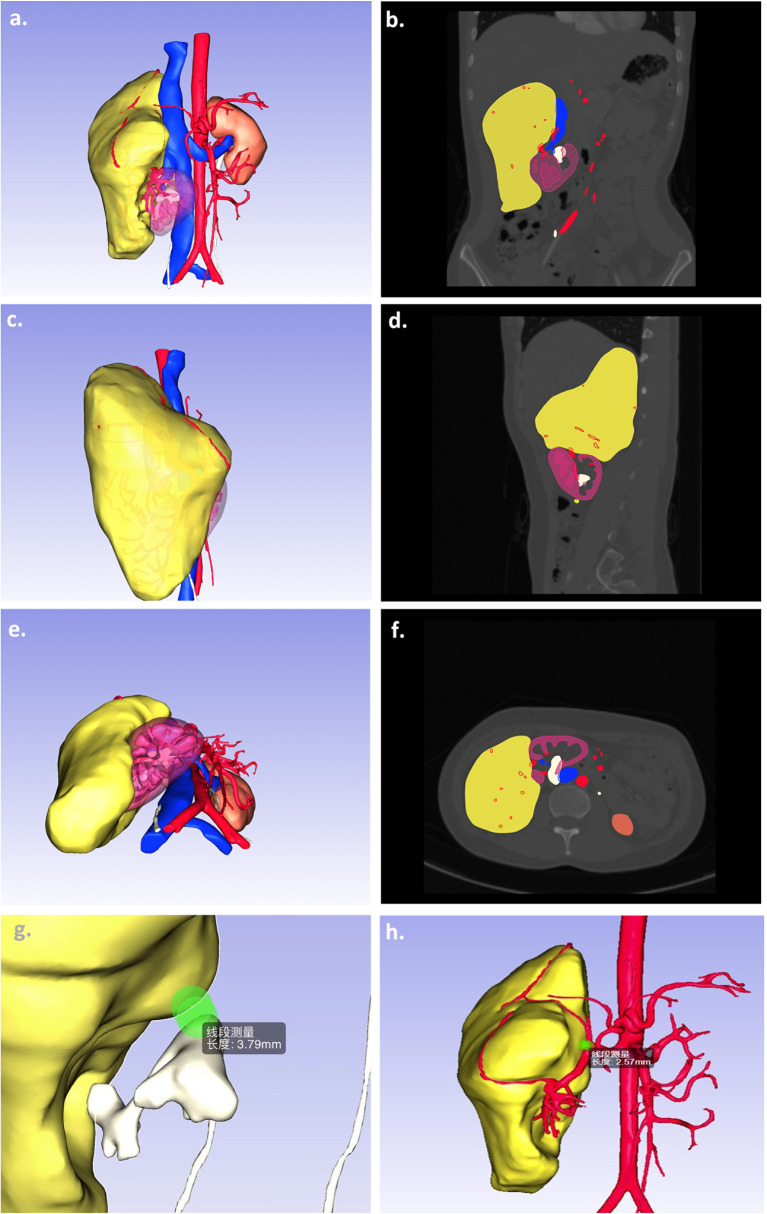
A sample of 3D segmentation of giant sporadic renal angiomyolipoma. Computed tomography scans of kidney tumors show the kidney (purple), tumor (yellow), arteries (red), veins (blue), and collecting system (white) segmentations as overlays (A, C, E). The images (B, D, F) present the corresponding 3D segmentations at different view positions. The other images demonstrated the important parameters, namely, the nearness of the tumor to collecting system (G) and the nearness of tumor to the second branch of renal artery (H).
